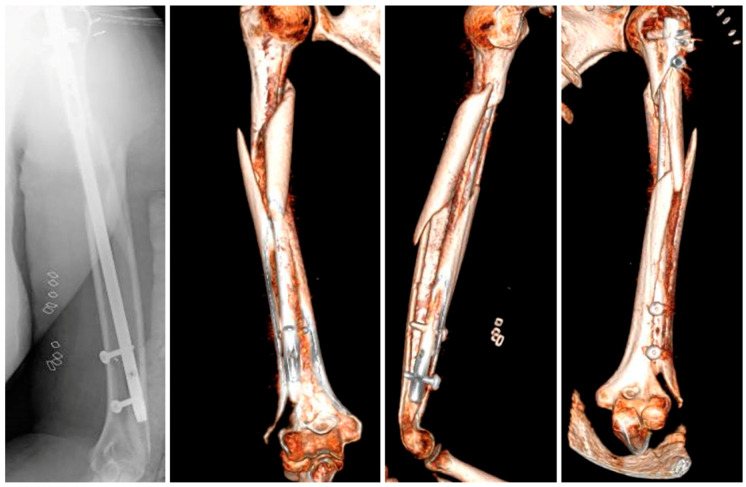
X-ray and 3D reconstruction of the peri-implant fracture.
